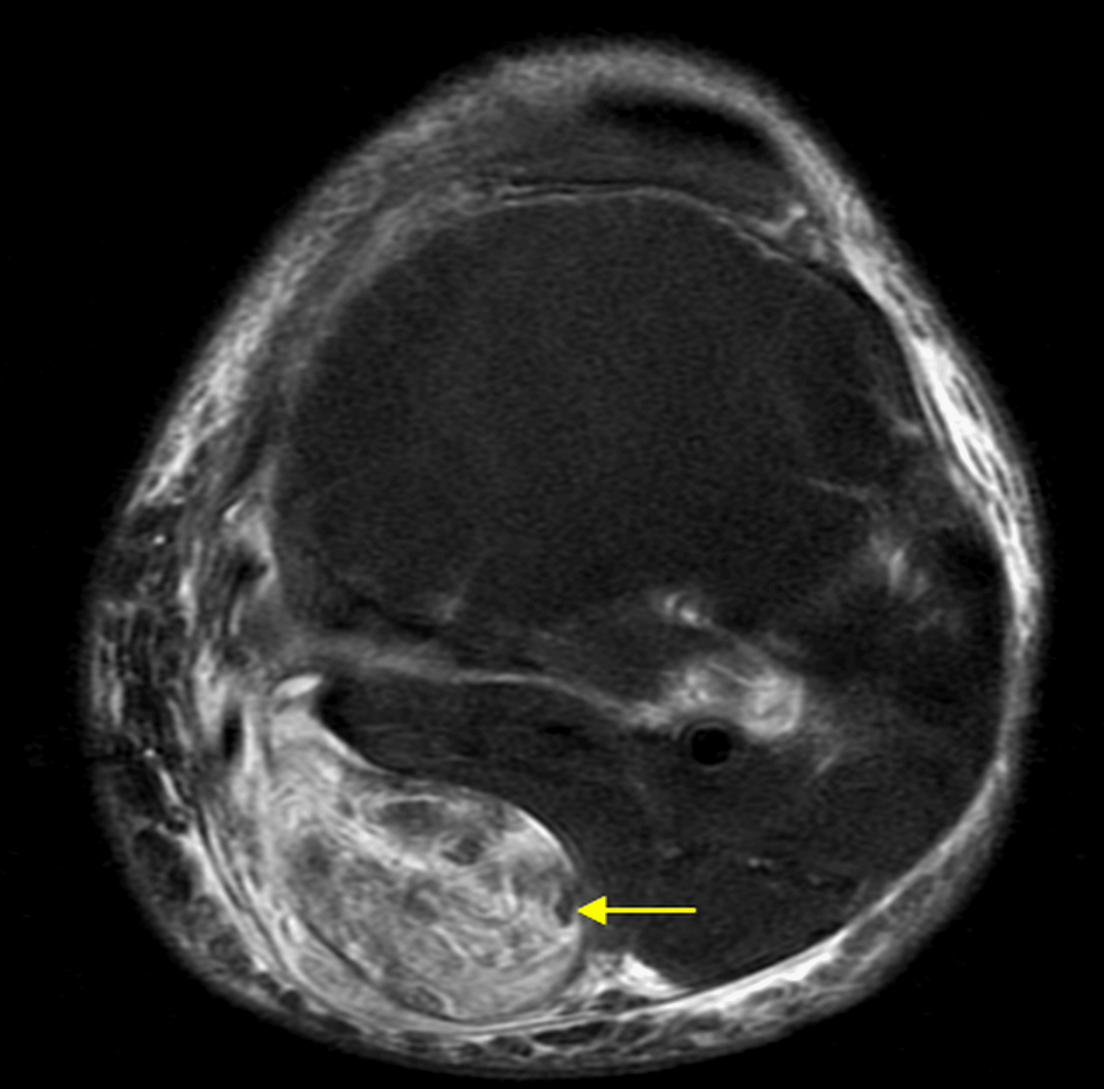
Axial MRI demonstrating a well-defined fluid-filled popliteal mass. The axial T2-weighted fat-suppressed image demonstrates a well-defined, hyperintense lesion (yellow arrow) with fluid signal intensity located in the popliteal fossa, exerting mild compression on the adjacent soft tissues.
